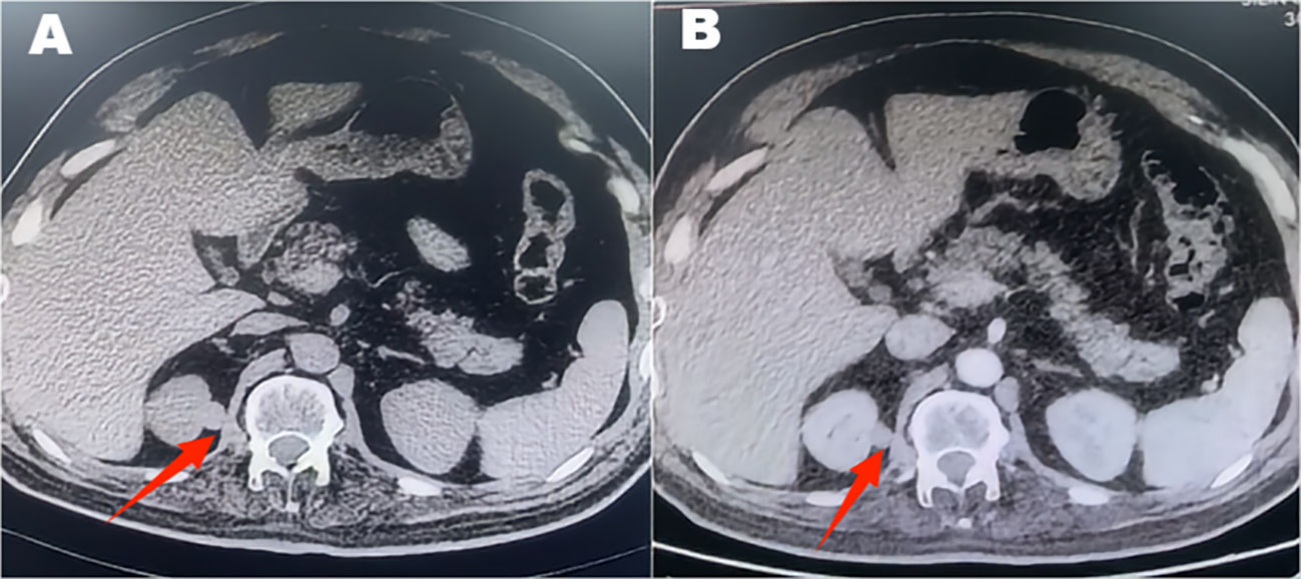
Contrast-enhanced CT demonstrates a well-defined, exophytic, hypodense nodule at the upper pole of the right kidney, showing minimal enhancement.
